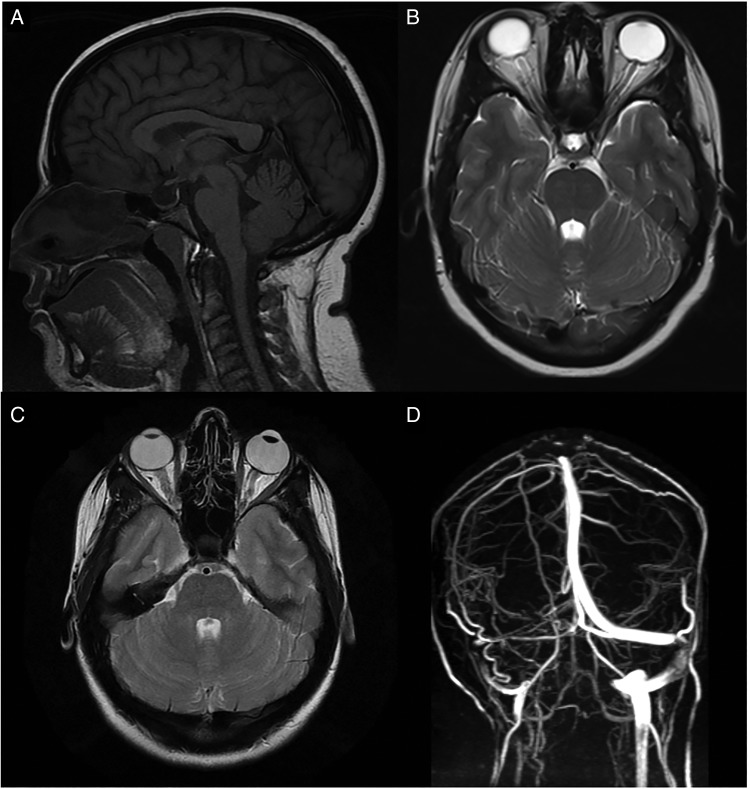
(A) MRI T1-weighted sagittal imaging demonstrating an empty sella (the pituitary gland has been flattened against the wall of the sella). (B) MRI T2-weighted axial image demonstrating flattening of the posterior globes at the insertion of the optic nerves, protrusion of the optic nerve head into the vitreous and increased fluid in the optic nerve sheath complex bilaterally. (C) MRI T2-weighted axial image demonstrating tortuosity (kinking) of the intraorbital optic nerve on the left with fluid in the associated optic nerve sheath complex. (D) MR venography (posterior view) demonstrating a longitudinal extensive left transverse sinus stenosis (extraluminal appearance).
